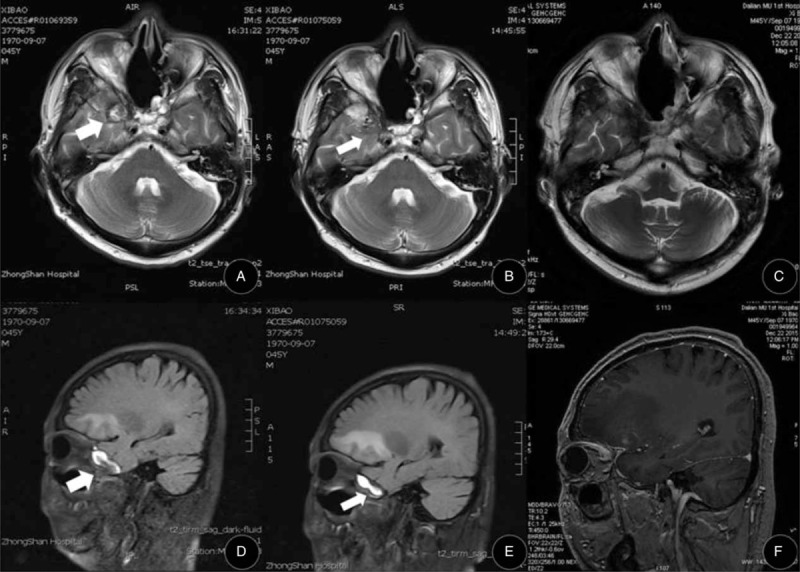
(A and D) Enhancement cerebral magnetic resonance imaging (MRI) showing the right temporal lobe space-occupying lesion (white arrows): (A) Horizontal T2 imaging; (D) Sagittal T2 imaging (size: 15.8 × 13.6 × 14.3 mm). (B and E) Five days after the cetuximab and sunitinib treatment, enhancement cerebral MRI shows the right temporal lobe space-occupying lesion (white arrows) is reduced in size with blurred boundary to peripheral tissue: (B) Horizontal T2 imaging; (E) Sagittal T2 imaging (size: 12.6 × 9.1 × 9.3 mm). (C and F) One month after treatment, cerebral MRI shows that the right temporal lobe space-occupying lesion has completely disappeared: (C) Horizontal FSE imaging; (F) Sagittal M3D/BRAVO imaging.
